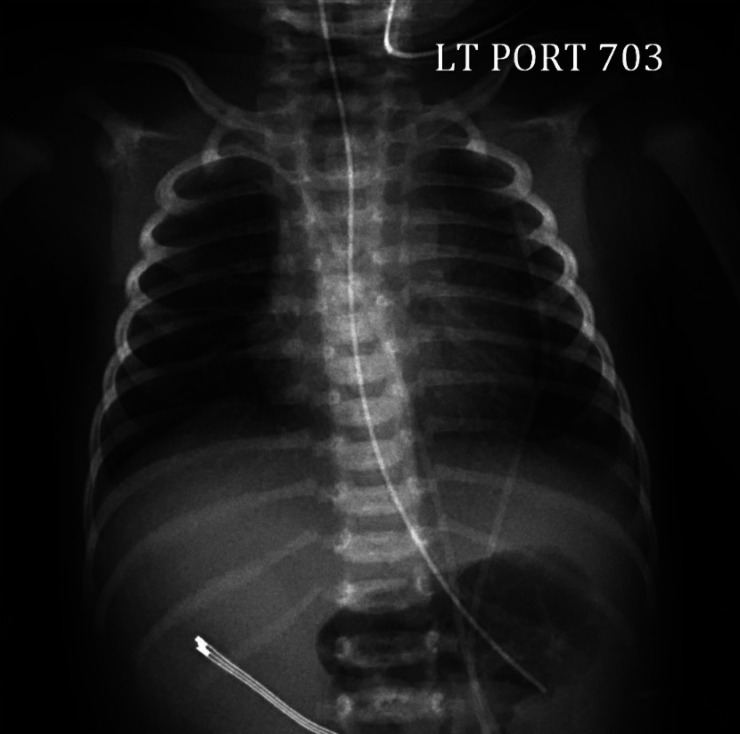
Chest X-ray of infant obtained at 90 min of life on CPAP.
